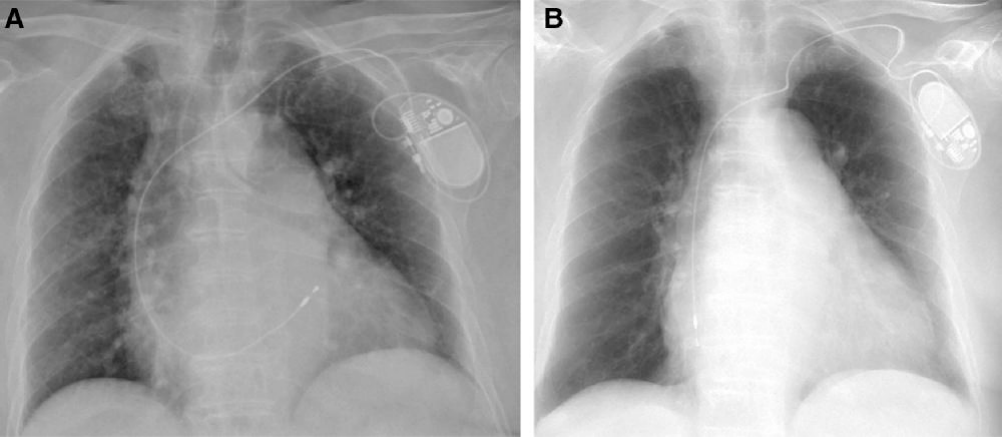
Case report 1. (*A*) Post-implant chest X-ray. (*B*) Chest X-ray—6 months later.
